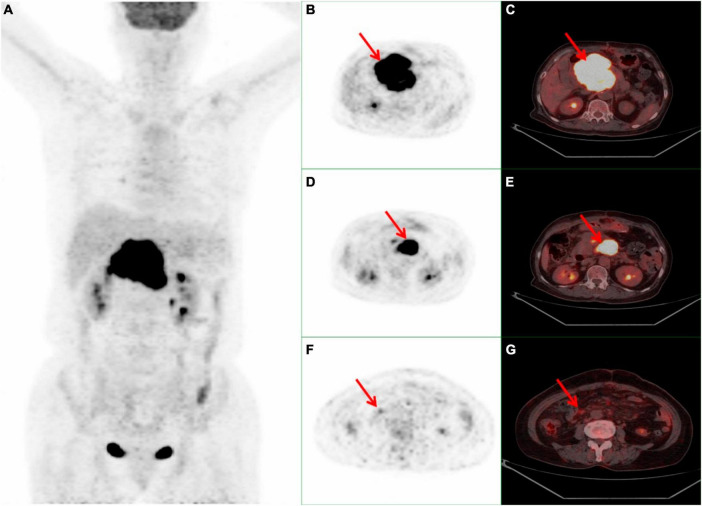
Whole body ^18^F-FDG PET/CT scan of a 70-year-old female with PPL. (A) MIP demonstrated a hypermetabolic lesion in the superior abdominal. (B,C) A 9.5 × 8.3 × 7.1 cm high FDG-avid irregular occupying at the head of the pancreas with a SUVmax 22.4 (red arrow). (D, E) Peripancreatic hypermetabolic enlarged lymph nodes (red arrow). (F, G) Suspicious mild hypermetabolic retroperitoneal lymph node with (red arrow). PPL, primary pancreatic lymphoma; ^18^F-FDG PET/CT, ^18^F-fluorodeoxyglucose positron emission tomography/computed tomography; MIP, maximum intensity projection; SUVmax, maximal standardized uptake value.
